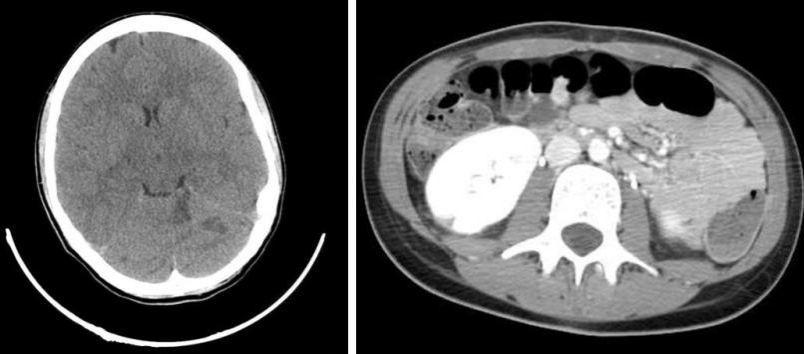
(a) Evidence of three contrast-enhancing lesions within the left cerebellar side on a CT central nervous system scan. (b) right kidney infarction.
